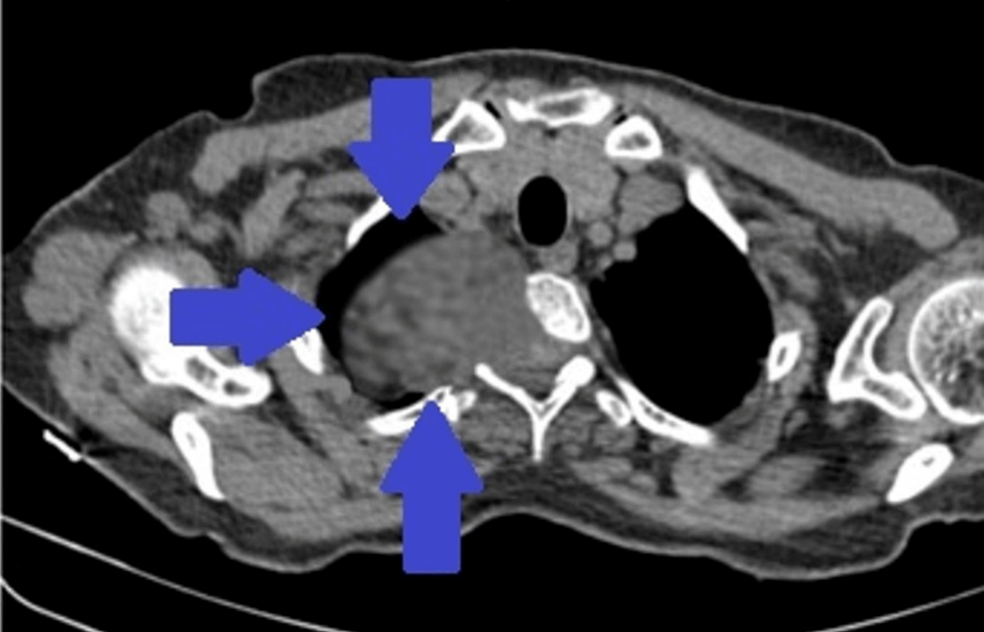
CT scan showing the large intrathoracic mass (blue arrows)
